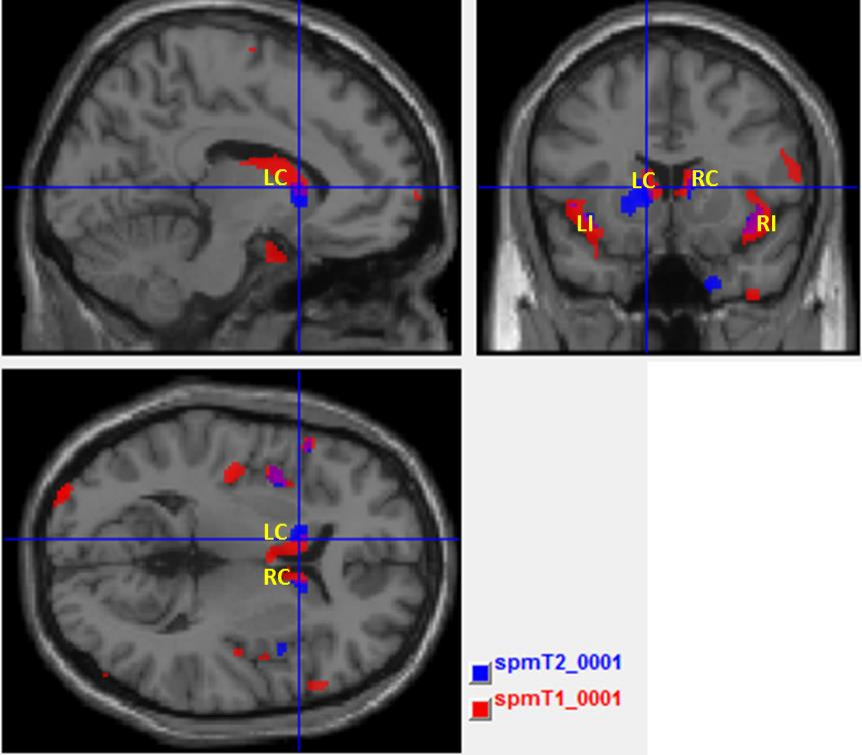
Overlapping of T1- (red clusters) and T2-weighted (blue clusters) univariate VBM analyses. The purple clusters correspond to the overlapped areas. LC – Left Caudate; RC – Right Caudate; LI – Left Insula; RI – Right Insula.
